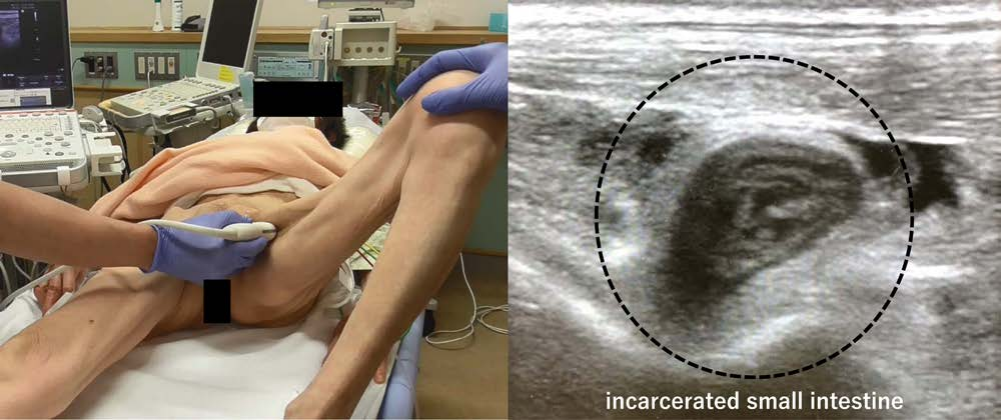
The surgeon locates and confirms the hernial sac between the adductor longus and the pubis. The assistant supports the patient to keep the hip joint flexed and rotated externally to ensure the hernial sac is well-visualized. The ultrasound image shows a dilated small intestine incarcerated in the hernial sac.
